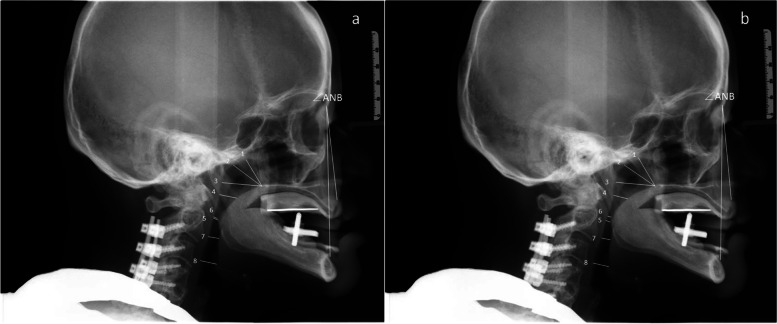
X-ray cephalometric lateral radiographs of the same patient as Fig. 1 at CRP and MP. a X-ray cephalometric lateral radiographs at MP. b X-ray cephalometric lateral radiographs at CRP. The numbers are measurement items of the upper airway as same as Fig. 3
